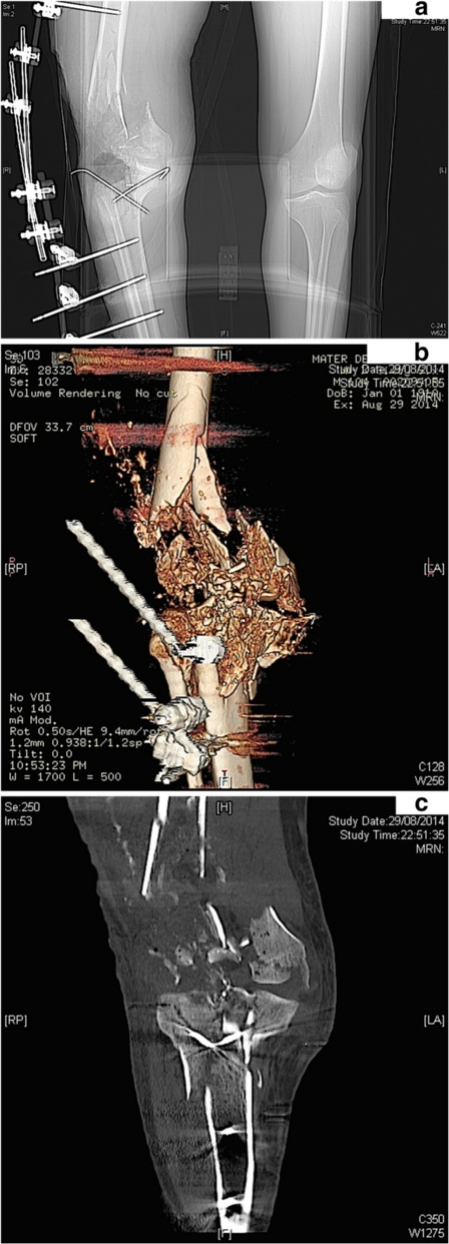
a DCO with a spanning external fixator holding what is left of the knee out to length. b-c CT 3D reconstruction (*above*) and coronal view (*below*) showing extent of knee trauma
